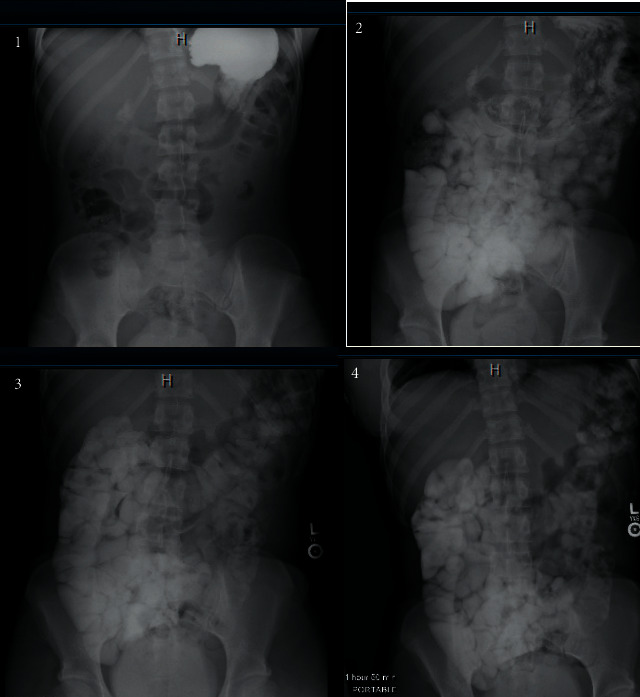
Upper gastrointestinal study with small bowel follow-through revealing no acute obstruction (radiographic images taken in series and are exhibited chronologically from 1–4.).
